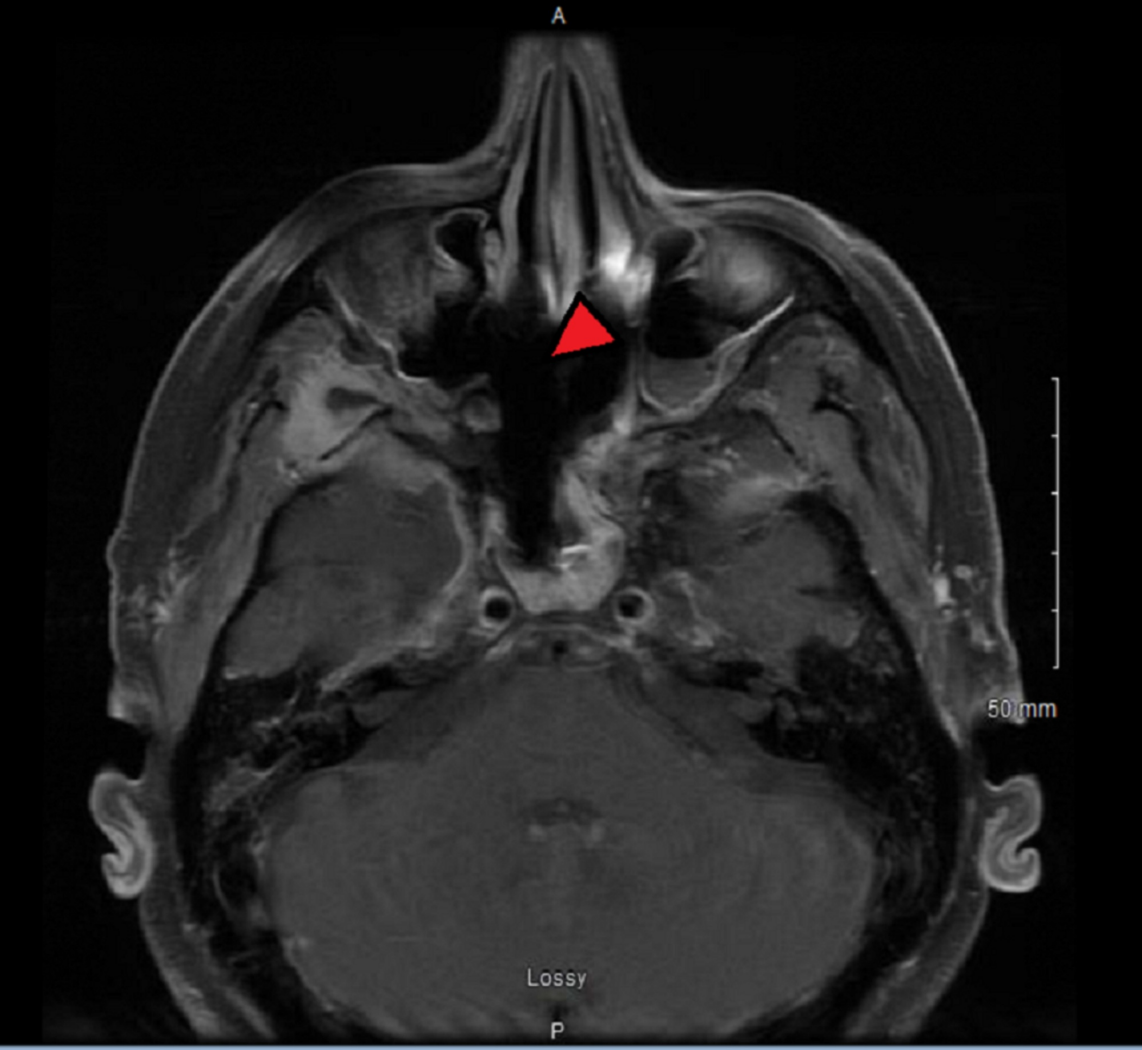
Magnetic resonance image of the axial section of the orbits and face Destruction of the right-side maxillary sinus can be seen (red arrow).
